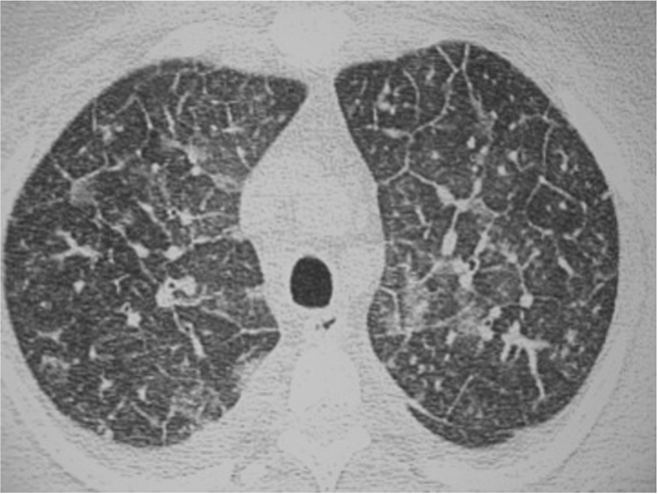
A 55 year-old female with pulmonary toxoplasmosis. Axial view of CT scan displays bilateral ground-glass opacities, interlobular and peribronchovascular smooth thickening and small bilateral pleural effusion.
